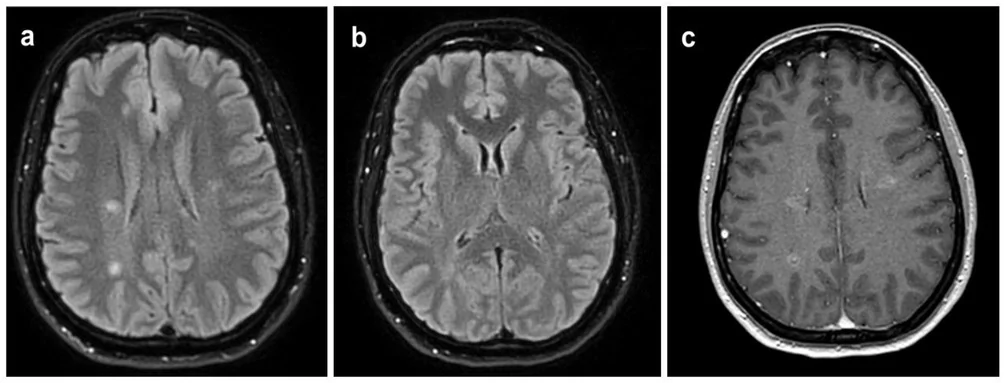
MRI brain axial FLAIR view obtained on day 4 after symptom onset, demonstrating multiple enhancing and non‐enhancing white matter lesions in the periventricular area (images a and b). Post‐contrast image included (image c). This was the patient's first MRI.
